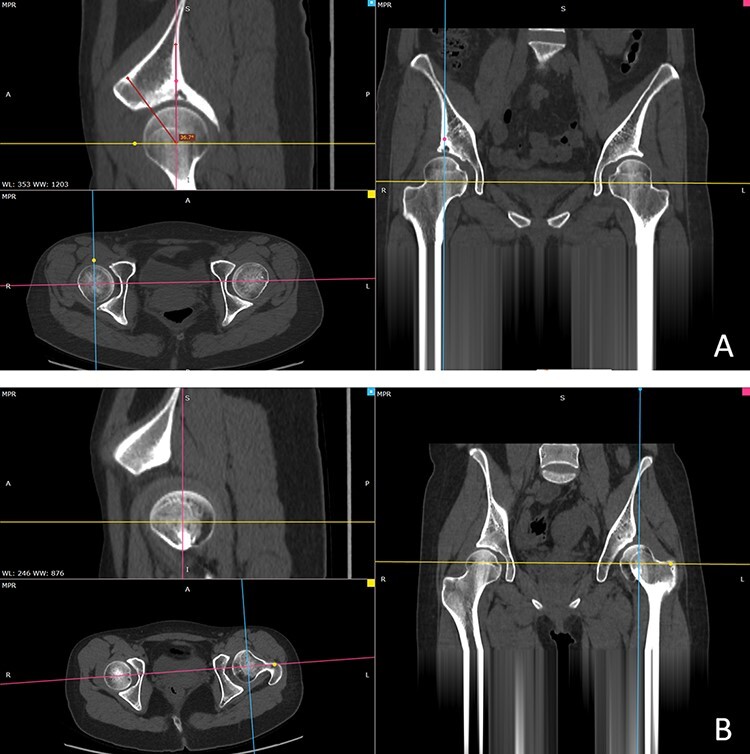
Measurement of the ACEA CT. (A) Adjust the observation plane in the multiplanar reconstruction interface to make the coronal and horizontal planes passing through the centers of both femoral heads. On the corrected sagittal plane, the angle between the vertical line and the line running through the femoral head and the anterior edge of the acetabulum is defined as the ACEA CT. (B) When the LCEA was less than zero, the acetabulum did not cover the femoral head on the sagittal plane, which goes through the center of the femoral head.
